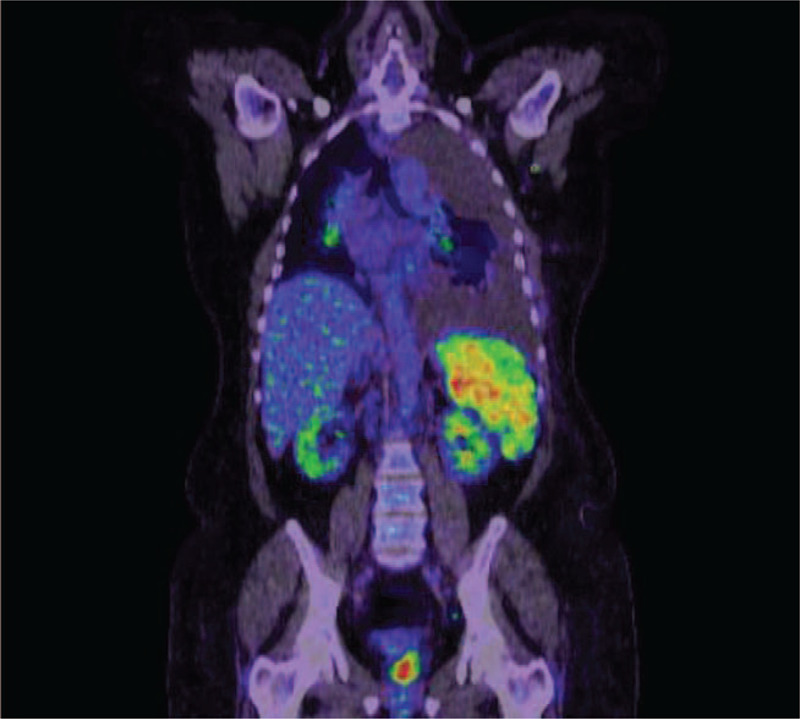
PET-CT scan showed increased pathological FDG uptake involving lymph nodes above and below the diaphragm in addition to the spleen. PET-CT = Positron emission tomography-computed tomography.
